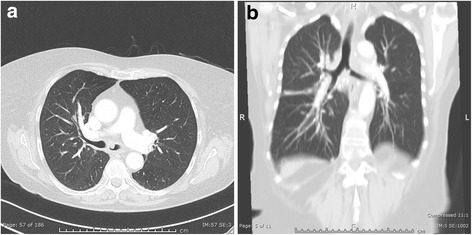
a, b Computed tomography with the polyp in the left main bronchus
